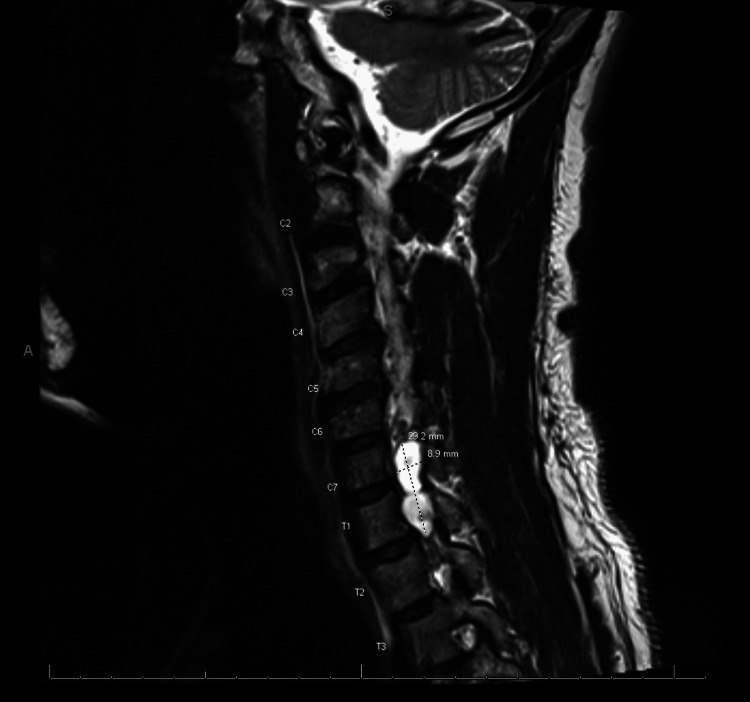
MRI with intravenous contrast of the cervical spine: right-sided pseudomeningocele in the cervical canal at C7 and T1. Non-visualization of the exiting C8 and T1 nerve root, which is consistent with a nerve root avulsion.
